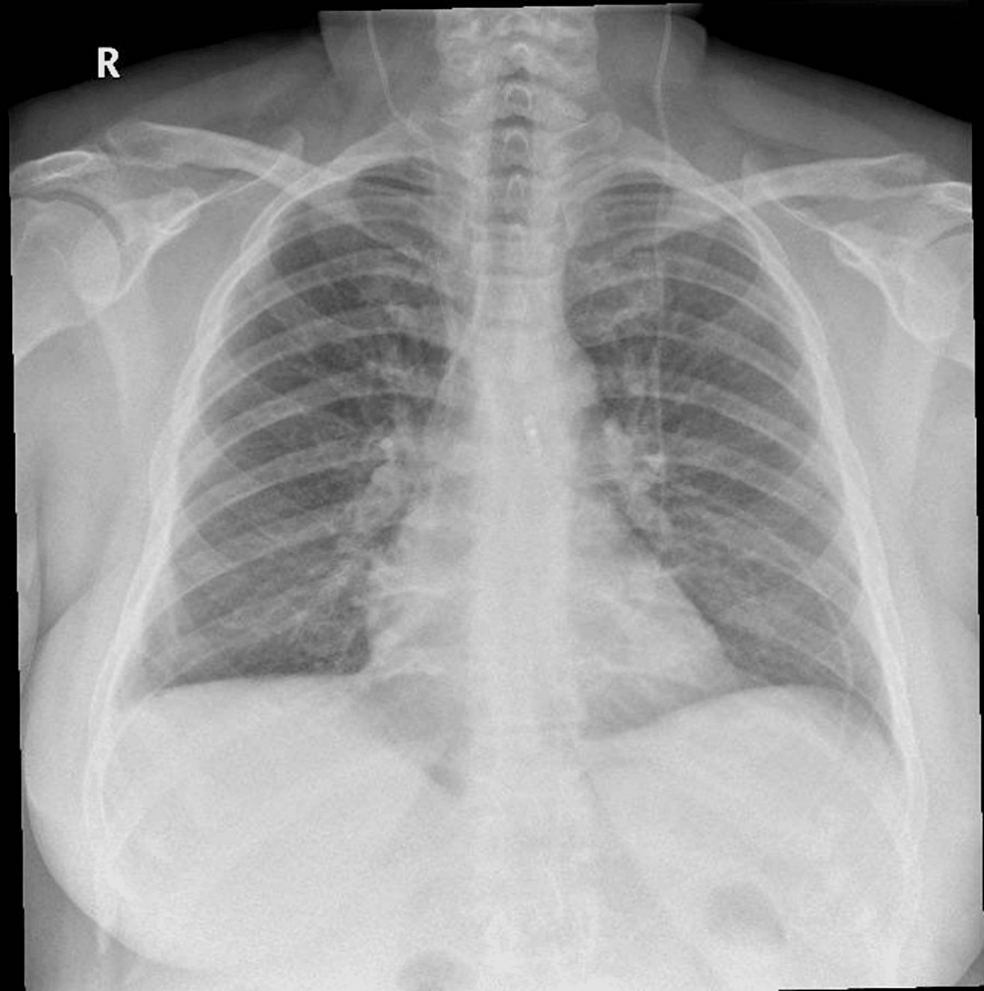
CXR done after shunt removal. Normal lungs and no pneumothorax noted CXR: chest radiography
